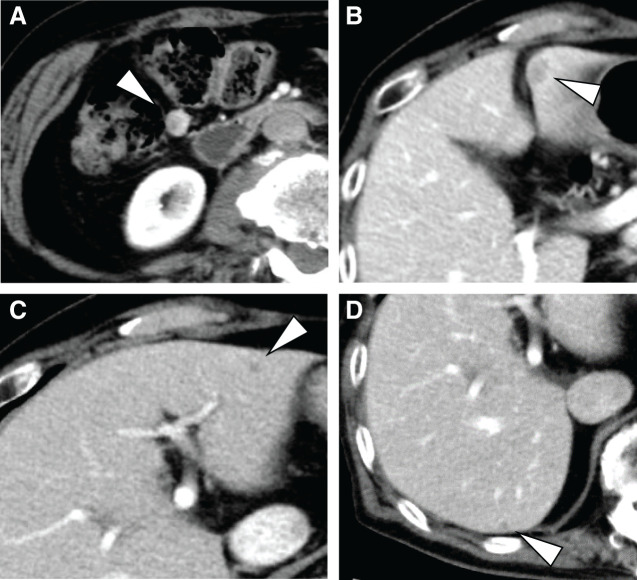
Abdominal CT at initial consultation. (A) Disseminated lesion near the duodenum. (B) Liver metastasis (S3). (C) Liver metastasis (S3). (D) Liver metastasis (S7).
